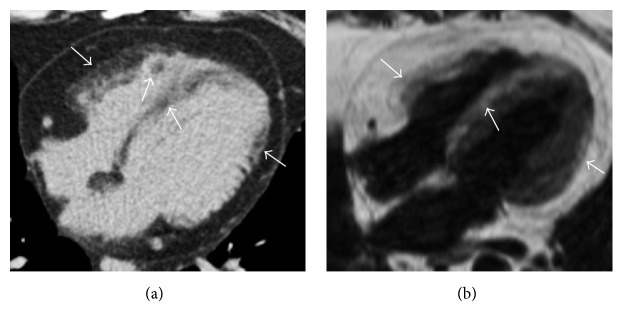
Axial thoracic noncontrast CT scan (a) shows multiple biventricular cardiac fatty foci with patchy pattern of appearance (arrows) in a patient with TSC, findings noted during an exam requested to evaluate pulmonary lymphangioleiomyomatosis. CMR with T1-weighted black blood four-chamber image (b) in the same patient confirms the presence of multiple corresponding fatty areas within the myocardium showing hyperintense signal (arrows).
